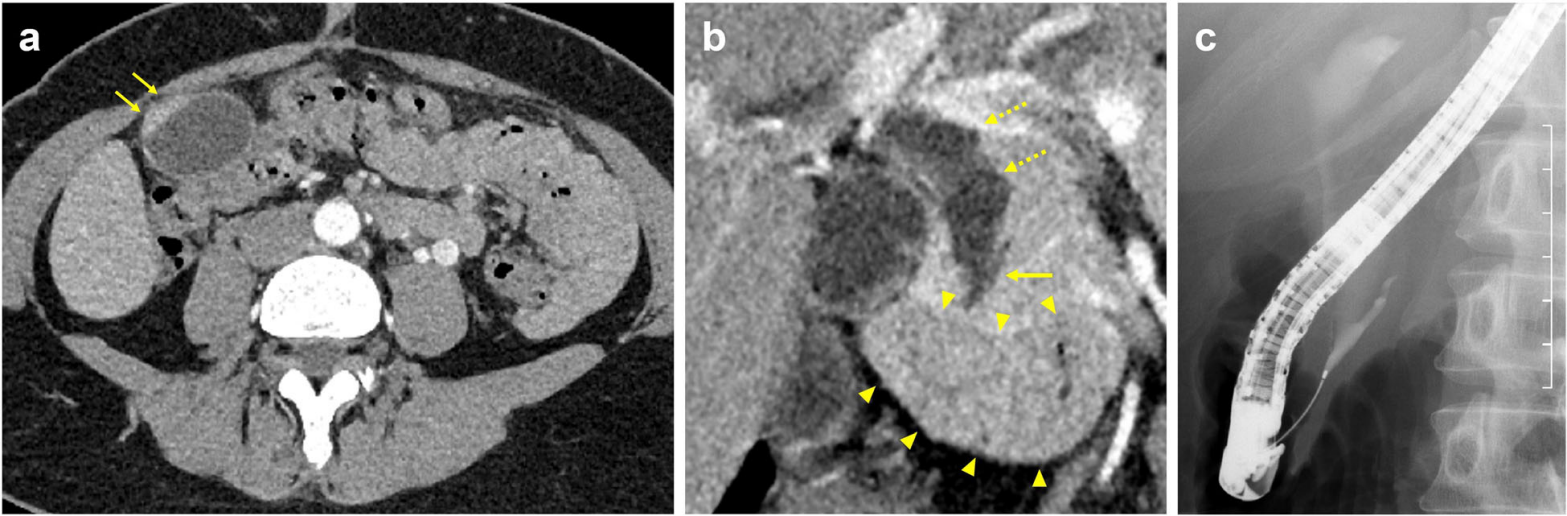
a Arterial-phase contrast computed tomography (CT) showing a mildly enhanced lesion in the gallbladder fundus (arrows). b Coronal image revealing that the dilated extrahepatic bile duct (EHBD) (dotted arrows) and the main pancreatic duct (arrow) joining into the EHBD at outside the duodenal wall (arrowheads). c Endoscopic retrograde cholangiopancreatography demonstrating the pancreaticobiliary maljunction
